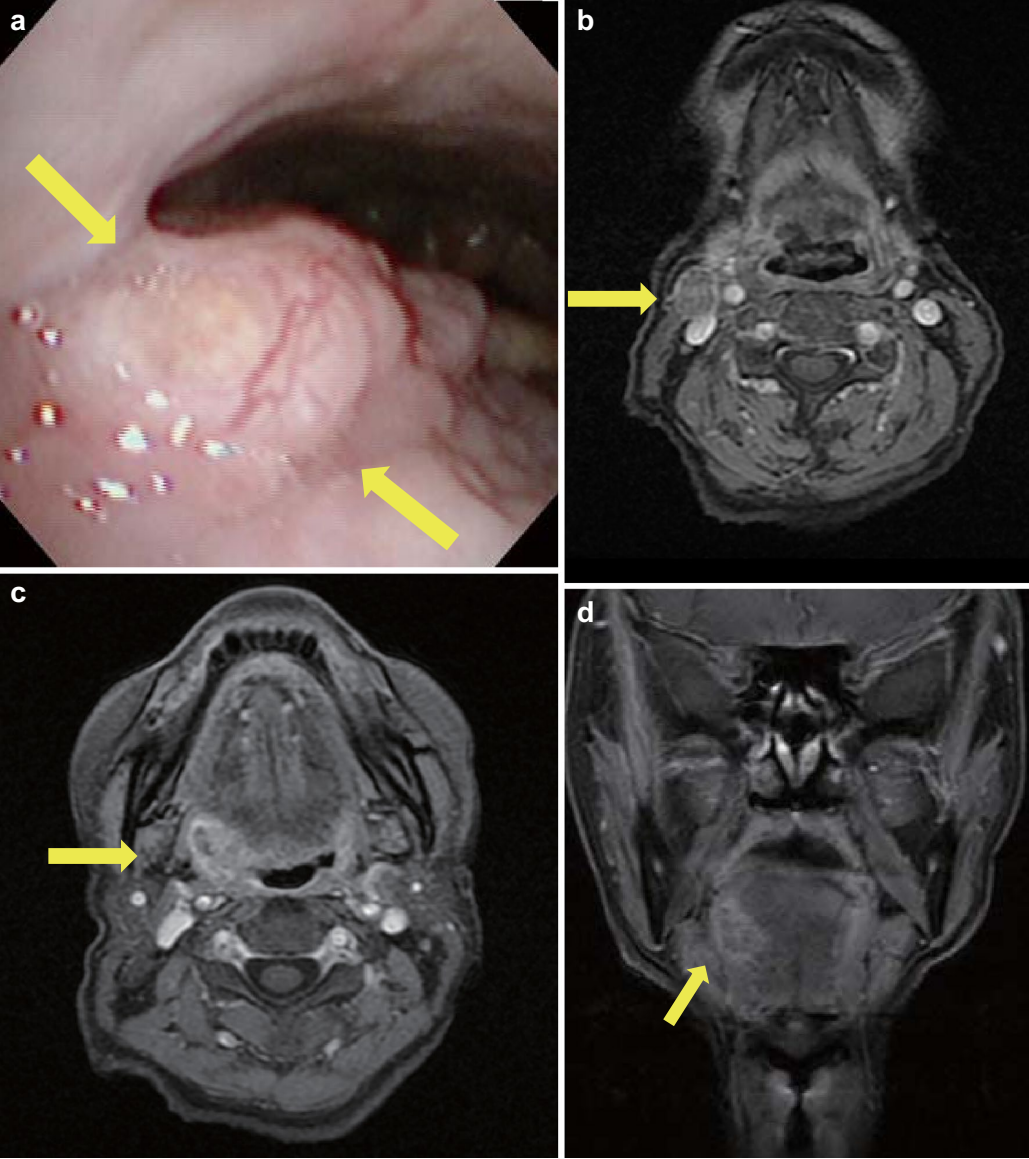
Case 1. a Oropharyngeal fiberscopic image. A tumor was observed in the right anterior wall of the oropharynx (*yellow arrow*). b Contrast-axial magnetic resonance imaging (MRI) scan of the neck before treatment. An enhanced metastatic tumor was observed in the right internal jugular node (*yellow arrow*). c Contrast-axial MRI scan of the neck before treatment. An enhanced primary tumor was observed in the right anterior wall of the oropharynx (*yellow arrow*). d Contrast-coronal MRI scan (*yellow arrow*)
